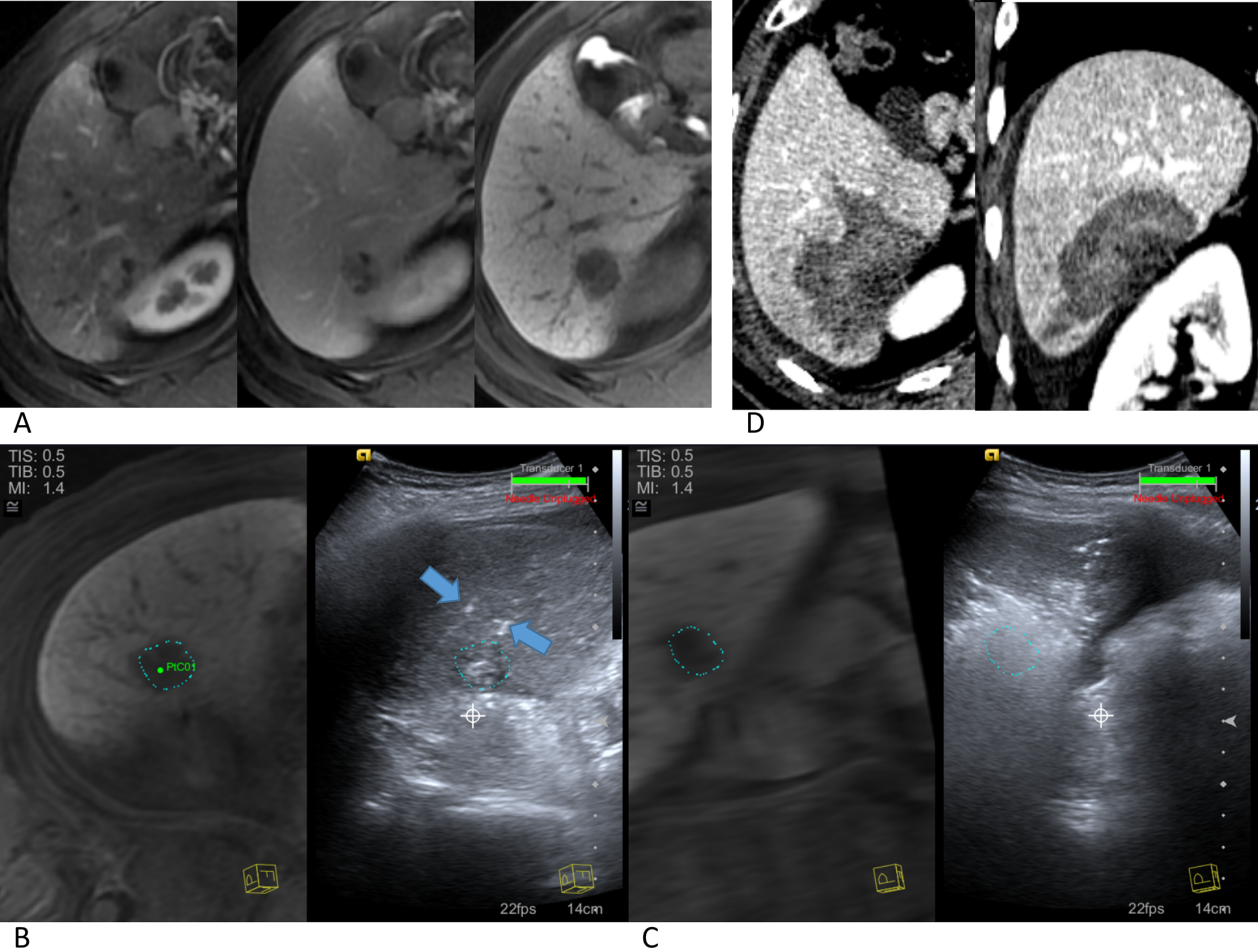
HCC in a 62-year-old man. (A) Axial MR images during arterial phase, portal phase and hepatobiliary phase after administration of gadoxetic acid show a 2.6 cm HCC with definitive arterial hypervascularization, venous washout, and hepatobiliary phase hypoenhancement. (B) Real-time US/MRI fusion image before ablation shows a slightly hyperechoic HCC on US image with virtual tumor margin and two electrodes (arrows) placed in the tumor and in the peritumoral area, respectively. (C) PostRFA US/MRI fusion image demonstrates that the virtual tumor margin suggesting the tumor location is covered by hyperechoic ablation zone with sufficient peritumoral margins. (D) Axial (left) and Coronal (right) immediate post-RFA CT images show complete ablation of the target tumor with sufficient peritumoral margins.
